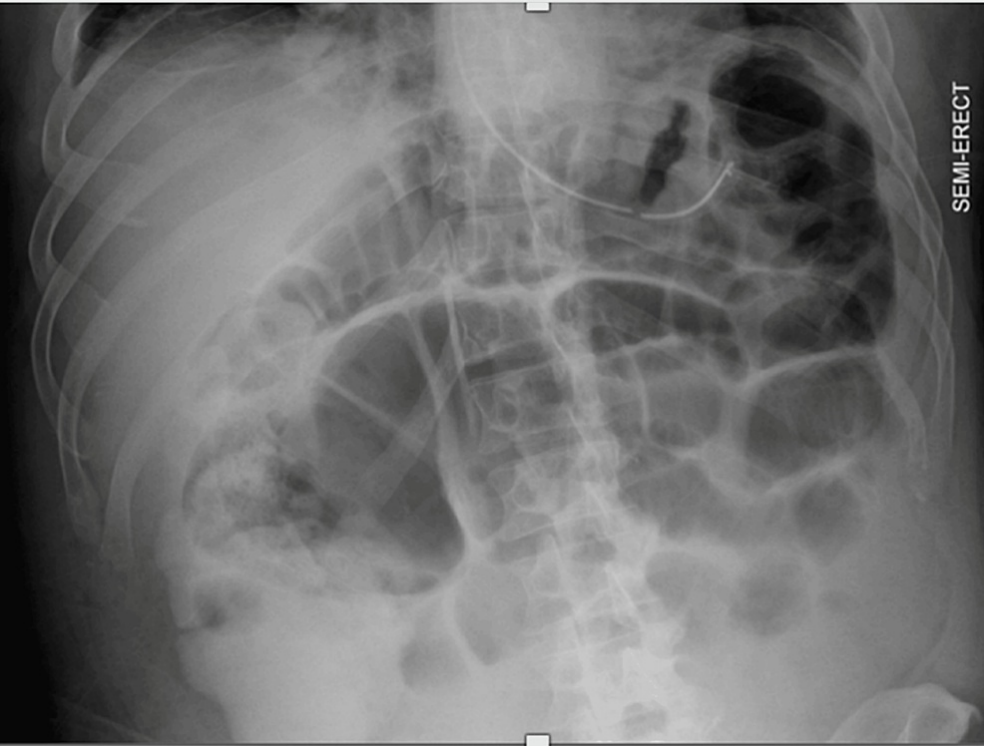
Case 2. X-ray of the thoracoabdominal high KUB revealing ongoing ileus.
